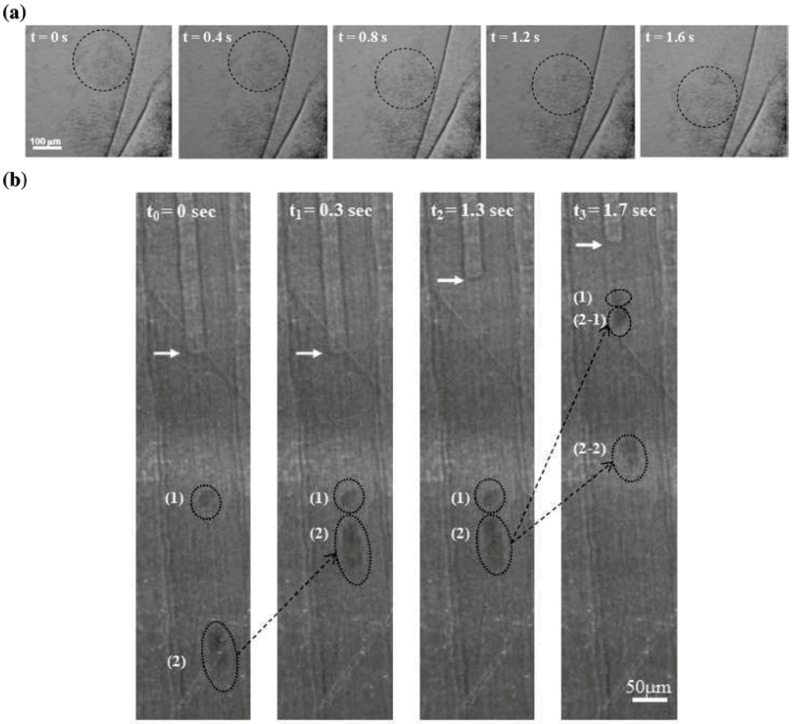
Application of AuNPs to the visualization of fluid dynamics in a living organism. (a) Time-dependent flow motions of the concentration-controlled hydrophilic AuNP aqueous solution captured by synchrotron X-ray imaging. (b) Four consecutive images showing the uptake and transport of hydrophilic AuNPs inside the xylem vessels of a rice leaf sheath visualized by synchrotron X-ray imaging. The arrow indicates the meniscus between water and air. (1) and (2) are initial positions of hydrophilic AuNP clusters in the sap. At *t*_0_ = 0 s and *t*_0_ = 0.3 s, only cluster (2) moves upward. At *t*_0_ = 1.3 s and *t*_0_ = 1.7 s, cluster (1) moves up, cluster (2) is simultaneously separated into two distinct clusters (2−1) and (2−2) with different velocity. The images are captured consecutively by the synchrotron X-ray source using a bending magnet. X-rays with a peak energy of 20.3 keV (8–30 keV range) are applied as a function of time without monochromator to obtain high energy [80]. Adapted by permission from The American Chemical Society.
